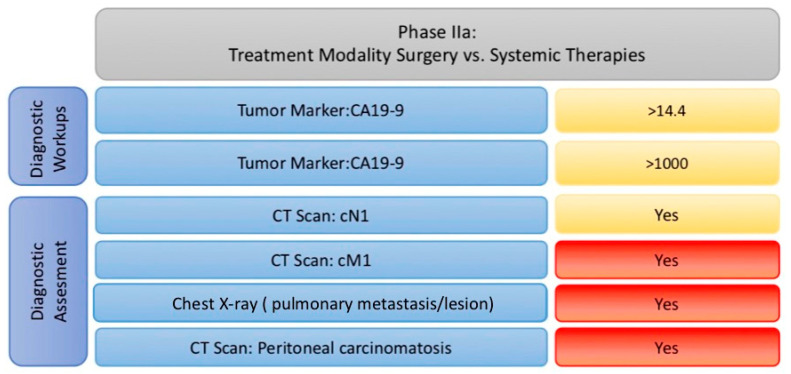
Factors implemented in phase IIa with their cut-offs adapted to traffic light model. (Abbreviations: CA 19-9: carbohydrate antigen; CT: computer tomography).
